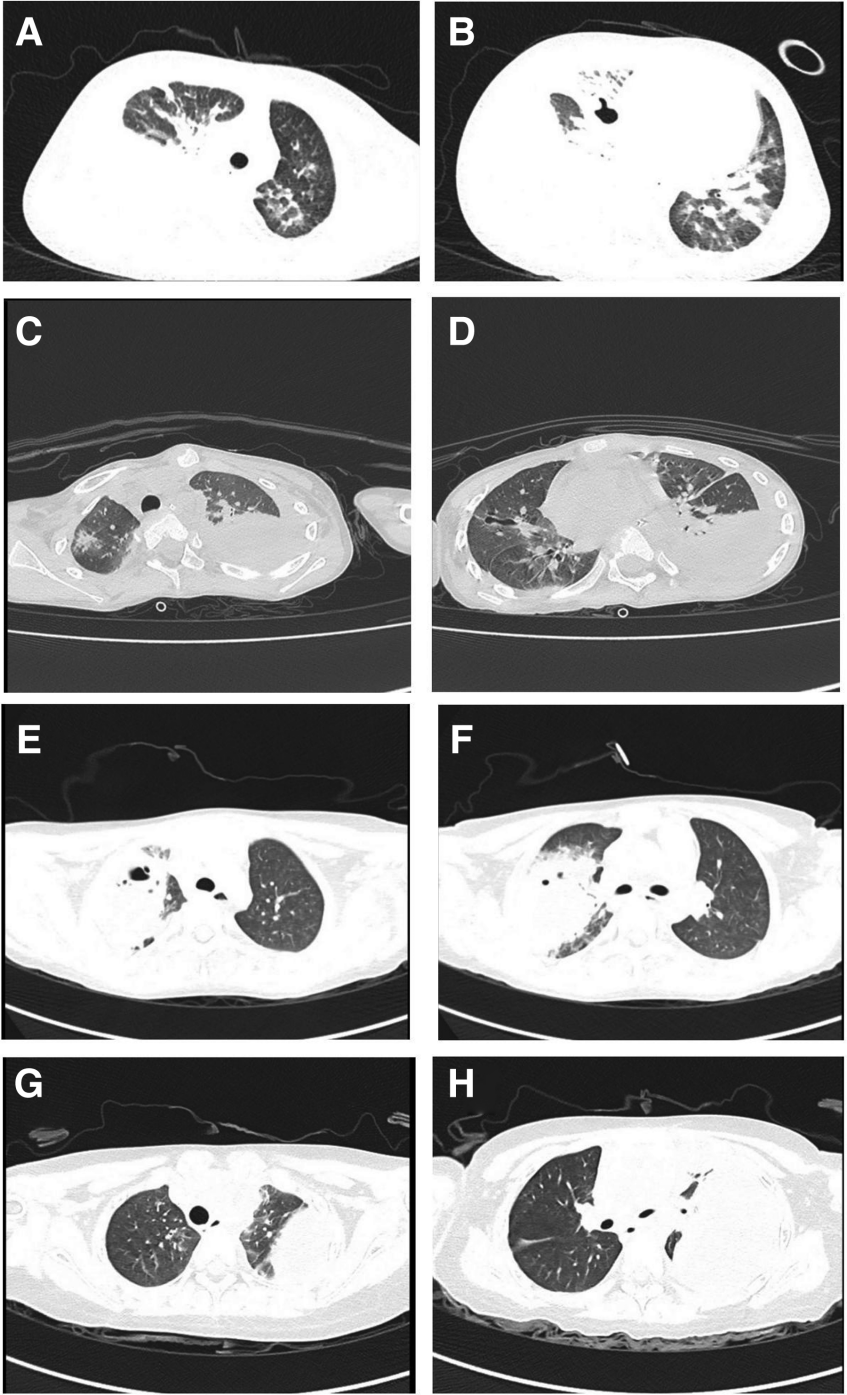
Pulmonary CT findings: (A,B) are the CT of case 1. (C,D) are the CT of case 2. (E,F) are the CT of case 3. (G,H) are CT of case 4.
